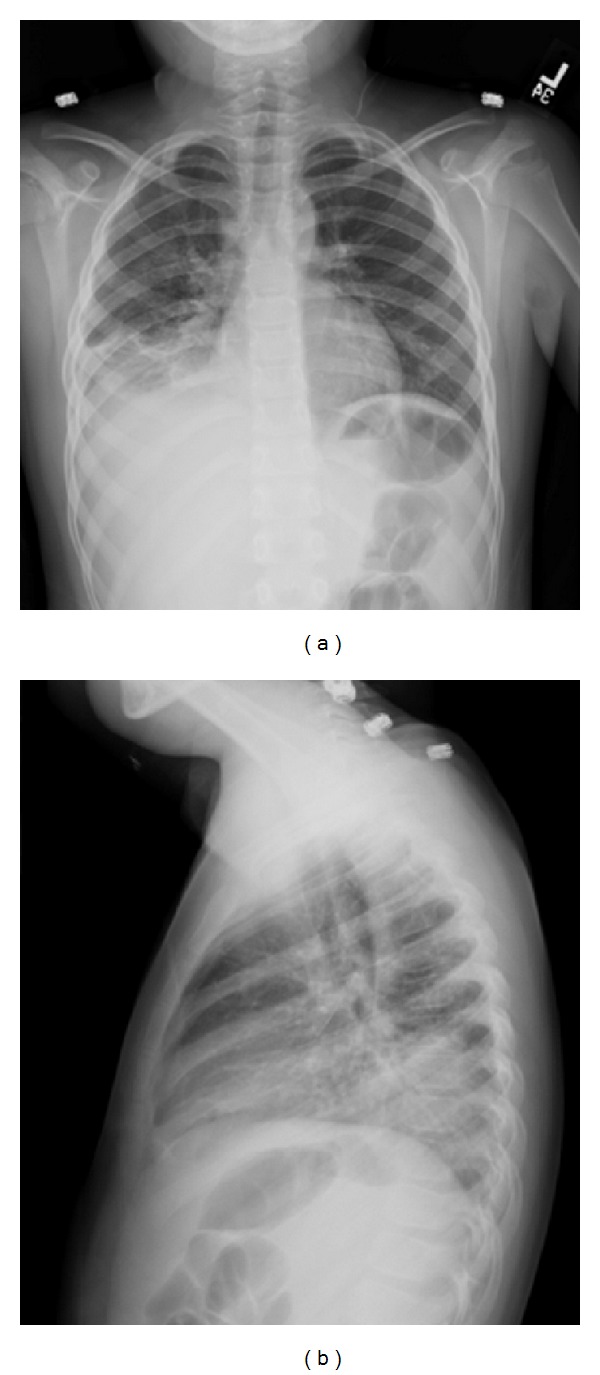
Chest radiographs during admission. Images demonstrating right lower lobe infiltrates. These films were taken at 9 years of age.
